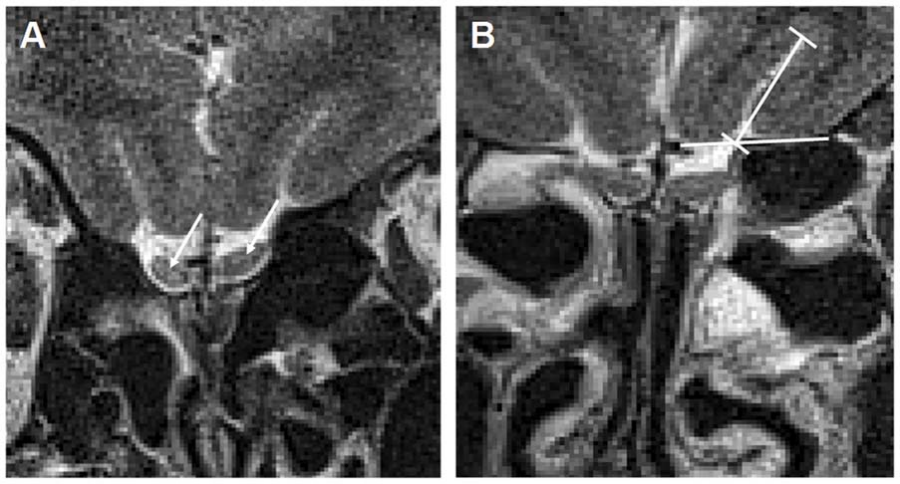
T2-weighted high-resolution coronal images of the olfactory bulb and sulcus olfactorius. Figure 1A and 1B show T2-weighted fast spin echo (FSE) sequences. In Figure 1A the white arrows indicate the normal dimensioned right and left bulb olfactorius. Figure 1B demonstrates the calculation of the olfactory sulcus (OS) depth. The distance of the deepest point of the OS was determined using a tangent line from the border of the gyrus rectus to the internal orbital gyrus.
